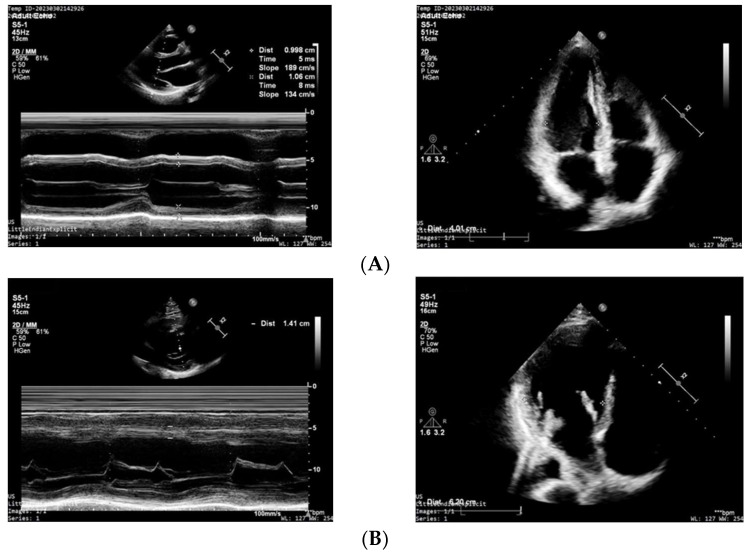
(A) Two-dimensional transthoracic echocardiographic image of a CABG patient with tHcy < 12 μM/L. Left panel: parasternal long axis view: normal thickness of interventricular septum (IVS) and posterior wall (PW) can be seen (10 mm). Right panel: Apical four-chamber view shows a normal left-ventricular end-diastolic diameter (LVED = 40 mm). (B) Two-dimensional transthoracic echocardiographic image of a CABG patient with tHcy > 12 μM/L. Left panel: Parasternal long axis view: considerably increased (14 mm) thickness of interventricular septum (IVS) and posterior wall (PW) can be seen. Right panel: Apical four-chamber view shows an increased left-ventricular end-diastolic diameter (LVED = 60 mm).
